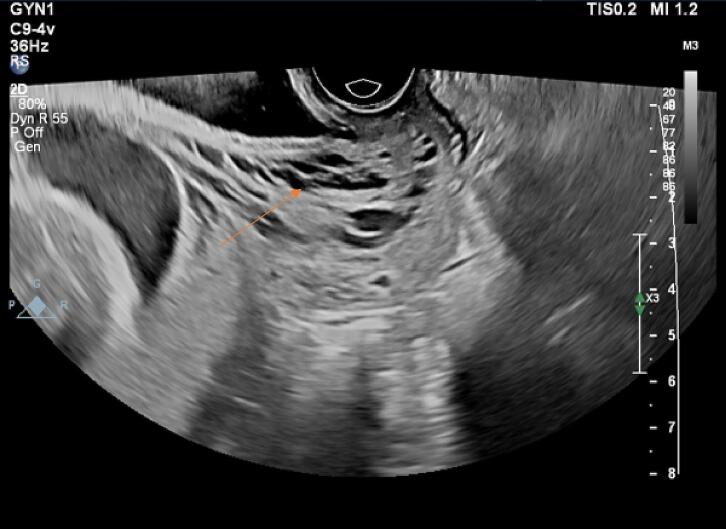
In the transverse-section of transvaginal sonography, blood vessels were fully extended to the circumference of the cervix (arrow).
